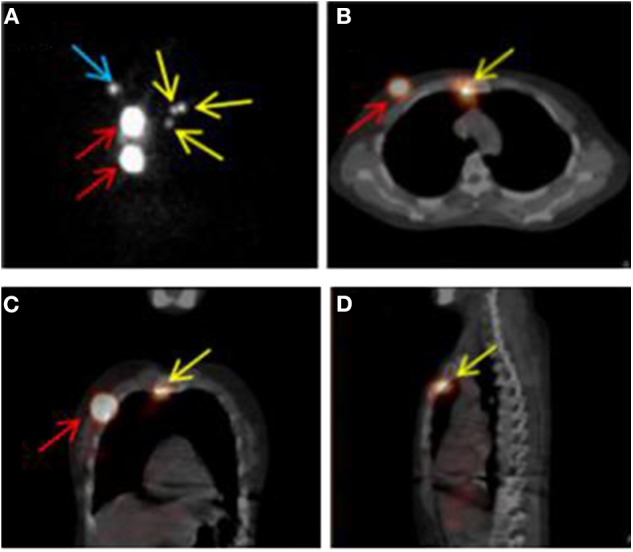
SPECT/CT combination imaging and positive sentinel lymph nodes. (A) SPECT image in anteroposterior position. (B–D) SPECT/CT fusion images. Red, yellow, and blue arrows indicate injection points, positive internal mammary sentinel lymph nodes, and axillary sentinel lymph node, respectively.
